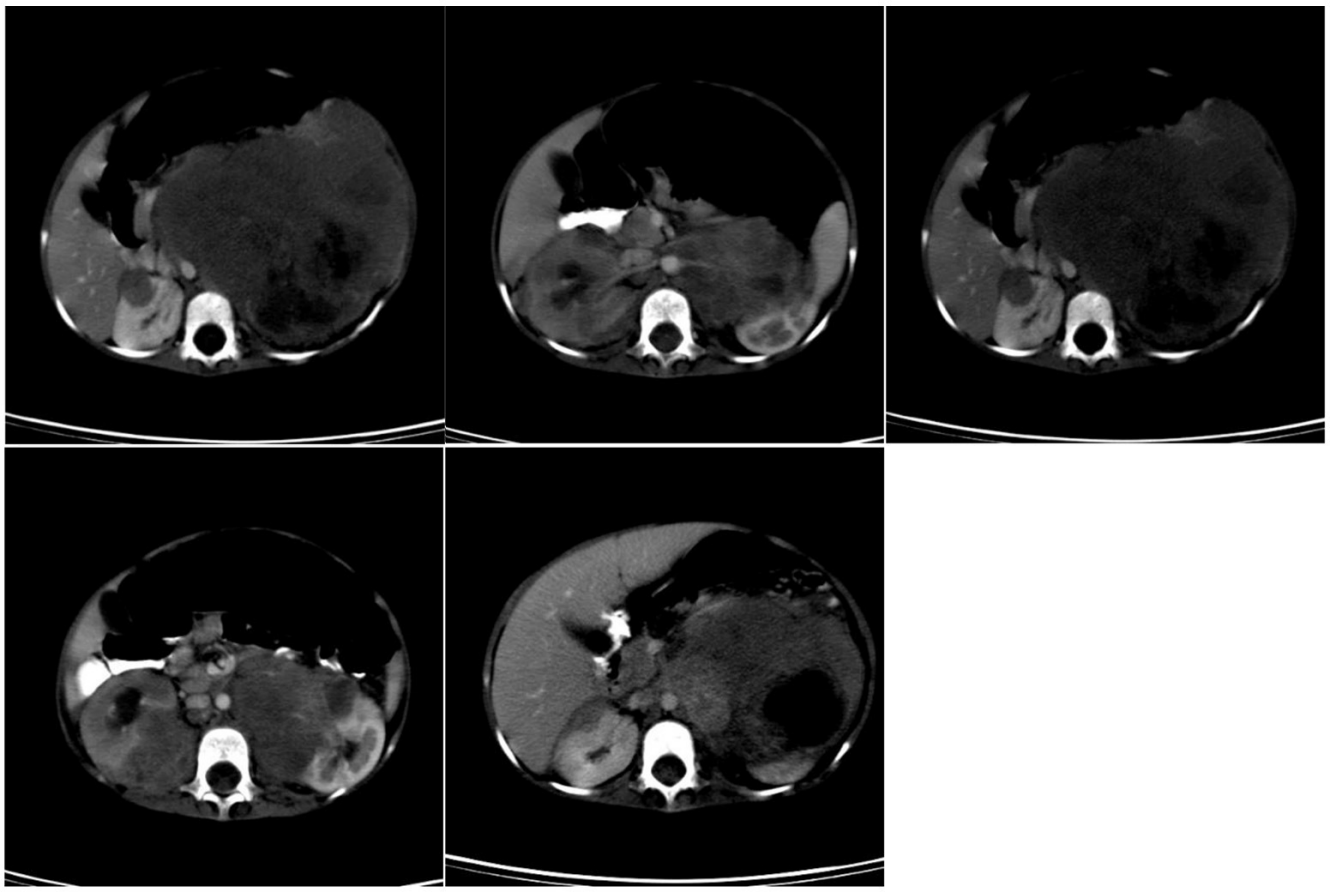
Preoperative CT scan of patient B.
